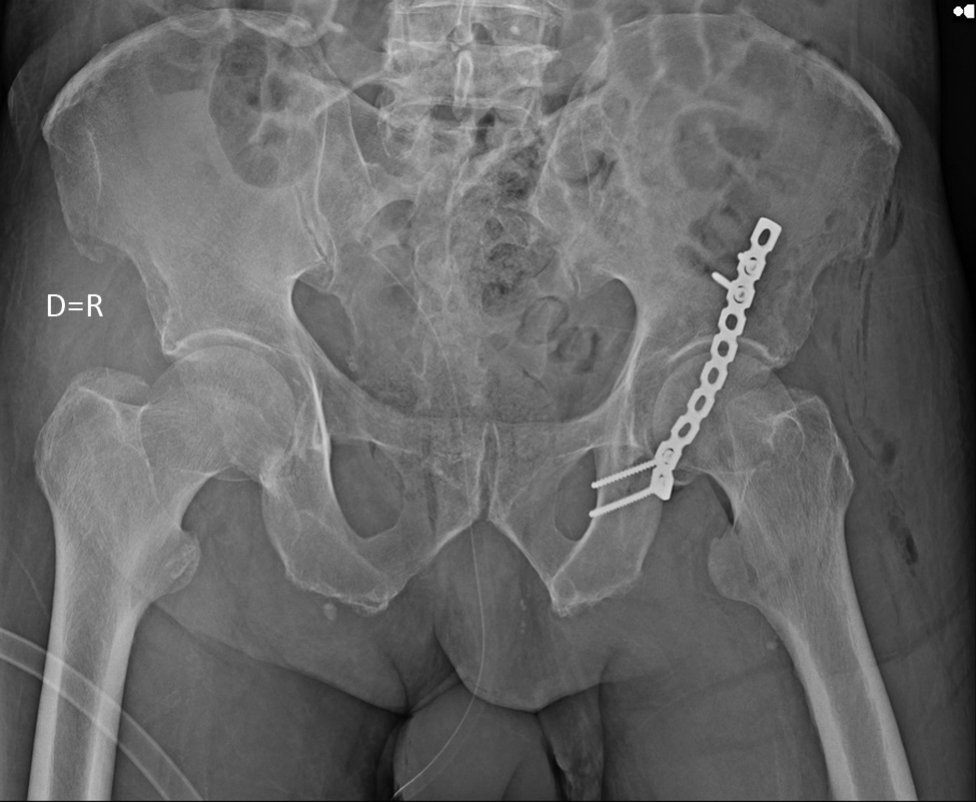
AP radiograph of the pelvis showing postoperative fracture of the posterior wall of the left acetabulum.
